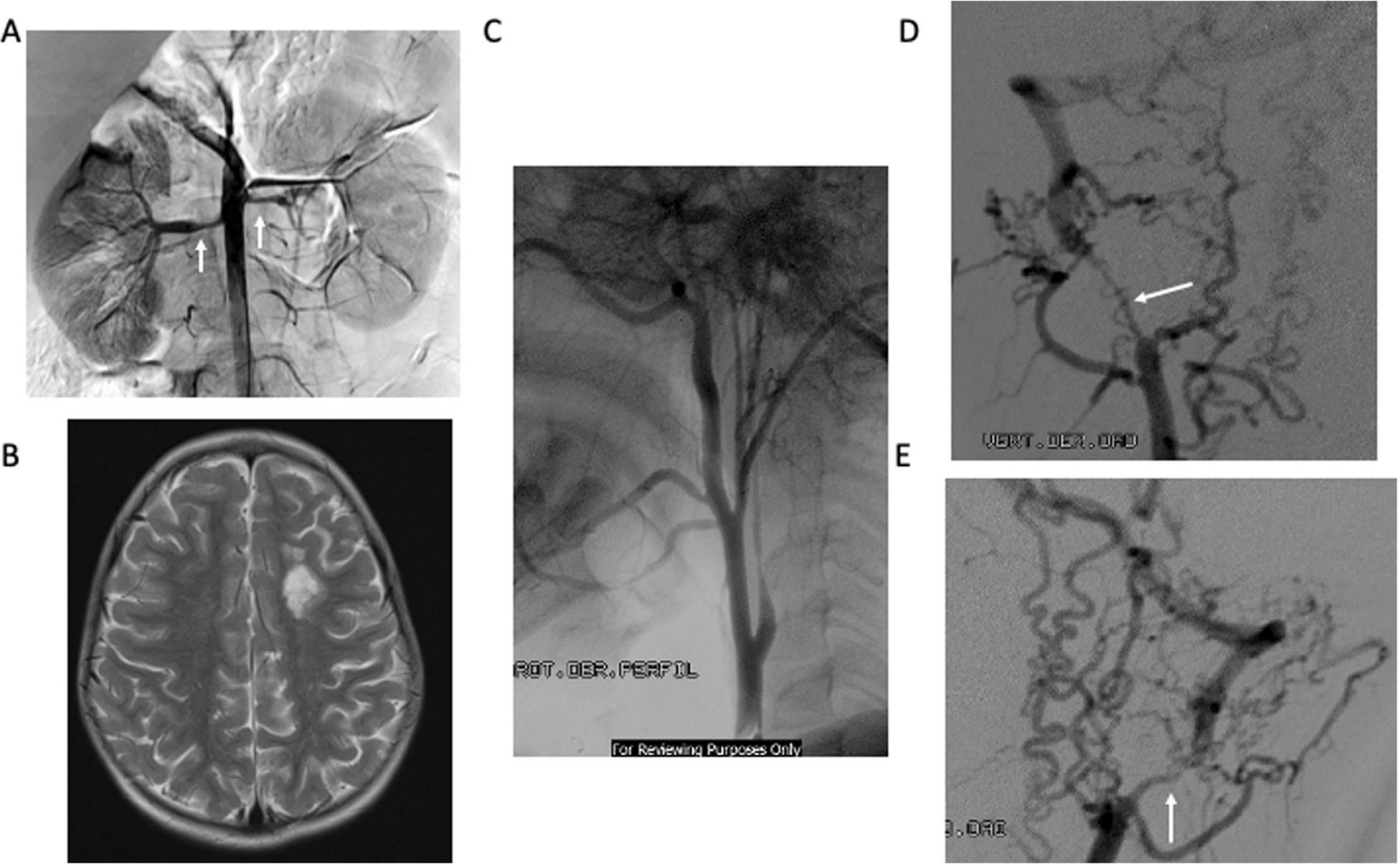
Scans results of patient. a Angiography image that shows bilateral renal artery stenosis (marked with arrows). b MRI—T2-weighted image showing left-hemisphere ischemic sequelae. c Angiography image showing right internal carotid stenosis. d Angiography image showing right vertebral artery stenosis. e Angiography image showing left vertebral artery stenosis
